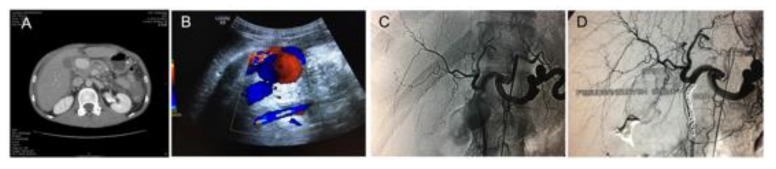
Diagnosis and treatment of a pseudo-aneurysm of the gastroduodenal artery in a patient with severe acute pancreatitis. (A) Abdominal angio-CT revealing a large contrast-enhanced mass, (B) characteristic swirl flow in abdominal ultrasound-Yin-Yang sign, (C) digital subtraction angiography (DSA) showing the large pseudoaneurysm projected over the gastroduodenal artery, and (D) DSA showing the post trans-arterial embolization of the gastroduodenal artery with multiple coils.
